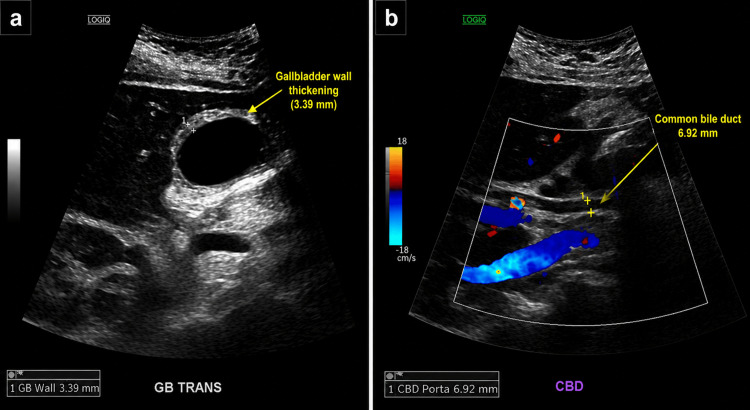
Abdominal ultrasound obtained during hospitalization. (A) Transverse gallbladder ultrasound demonstrating mild gallbladder wall thickening measuring 3.39 mm without visualized cholelithiasis. (B) Color Doppler ultrasound demonstrating a mildly prominent common bile duct measuring 6.92 mm without definite obstructive biliary dilation.
